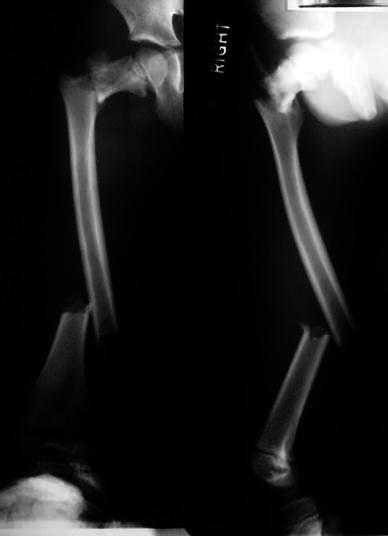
Boy seven years and ten months of age: anteroposterior and lateral radiographs reveal a right intertochanteric fracture (type IV in Delbet’s classification; AO classification type III) and an ipsilateral lower one-third fracture of the femoral shaft
